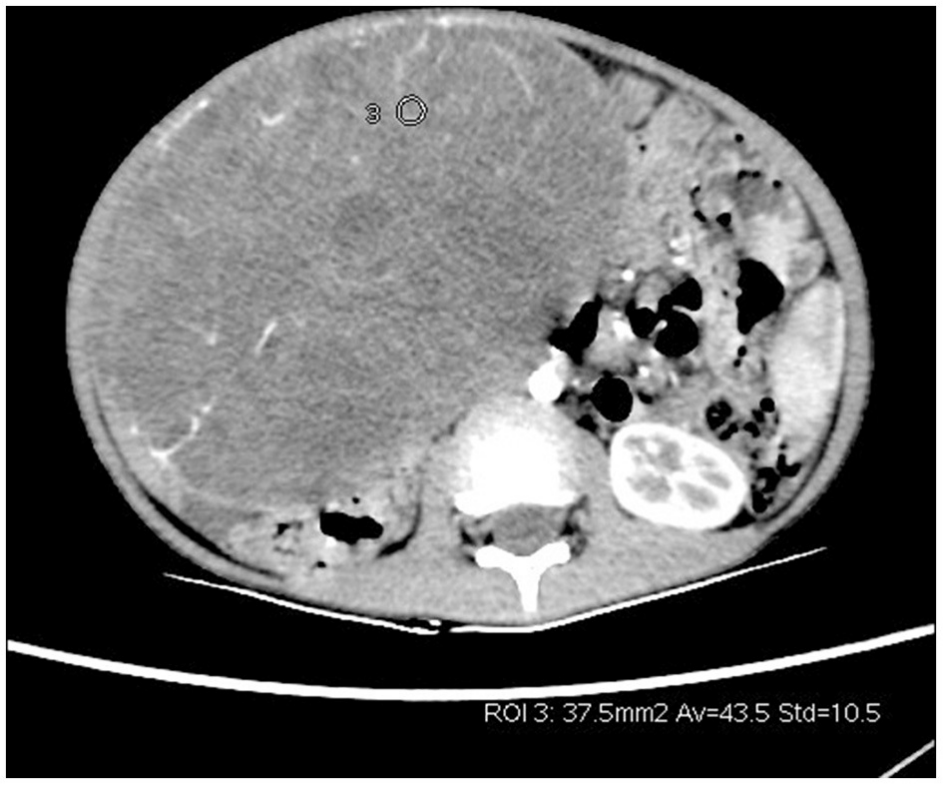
Enhanced CT of the arterial phase of a giant solid-cystic lesion in the right lower abdomen with abundant tumor vascularity.
